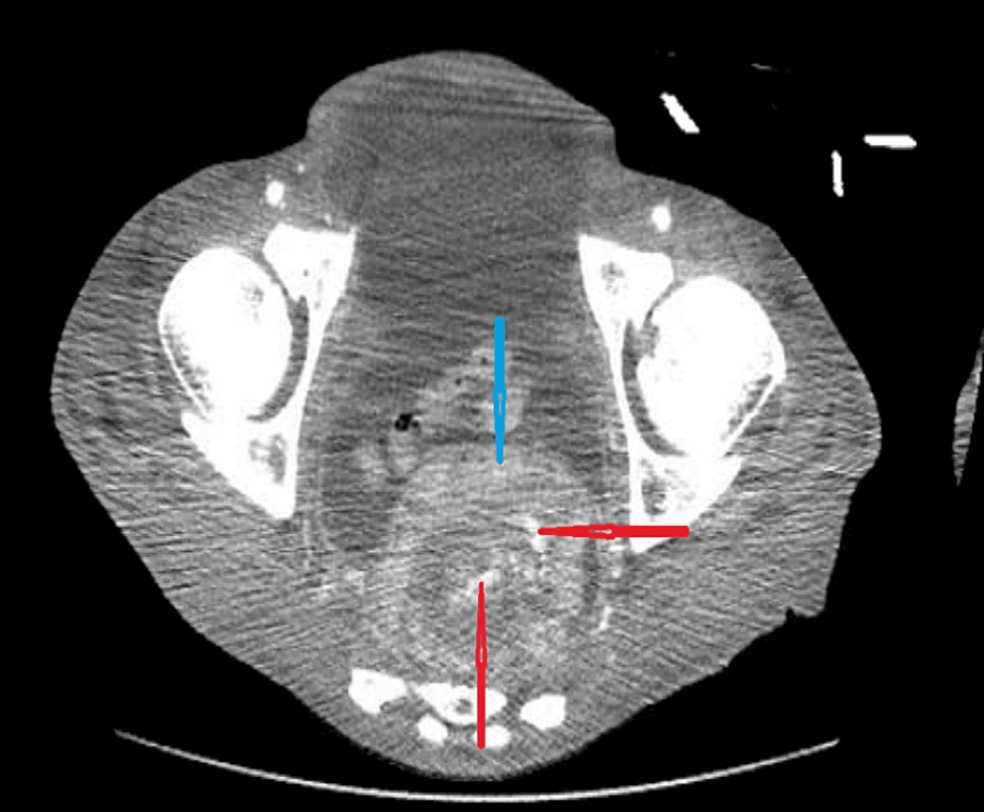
Computed tomography (CT) of the abdomen and pelvis in axial section shows abnormal moderate nodular rectosigmoid colonic wall thickening (blue arrow) with angiogenesis (red arrow)
